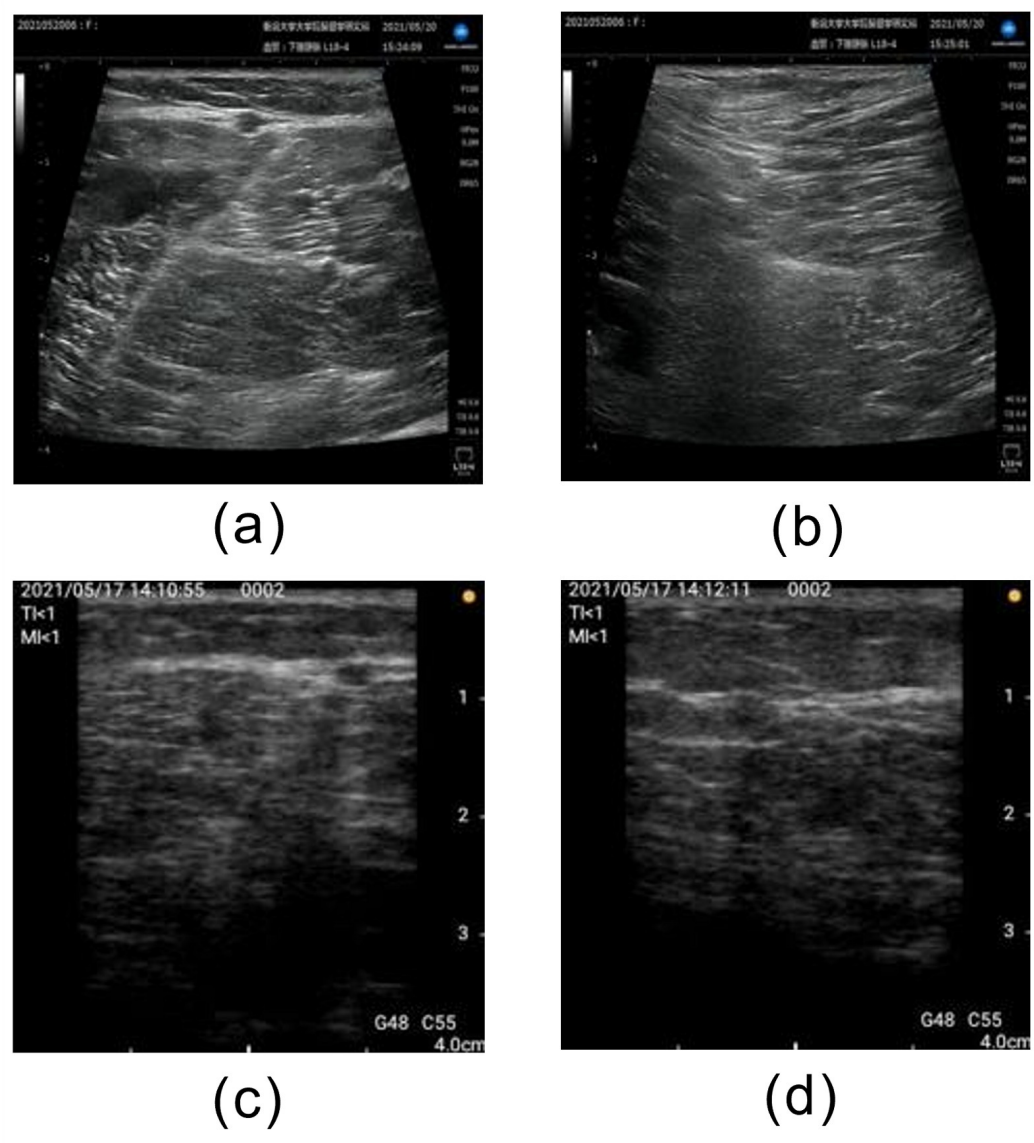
Examples of correctly classified unsatisfactory images. (a) Short axis acquired by stationary ultrasonography equipment, (b) Long axis acquired by stationary ultrasonography equipment, (c) Short axis acquired by portable ultrasonography equipment, and (d) Long axis acquired by portable ultrasonography equipment.
